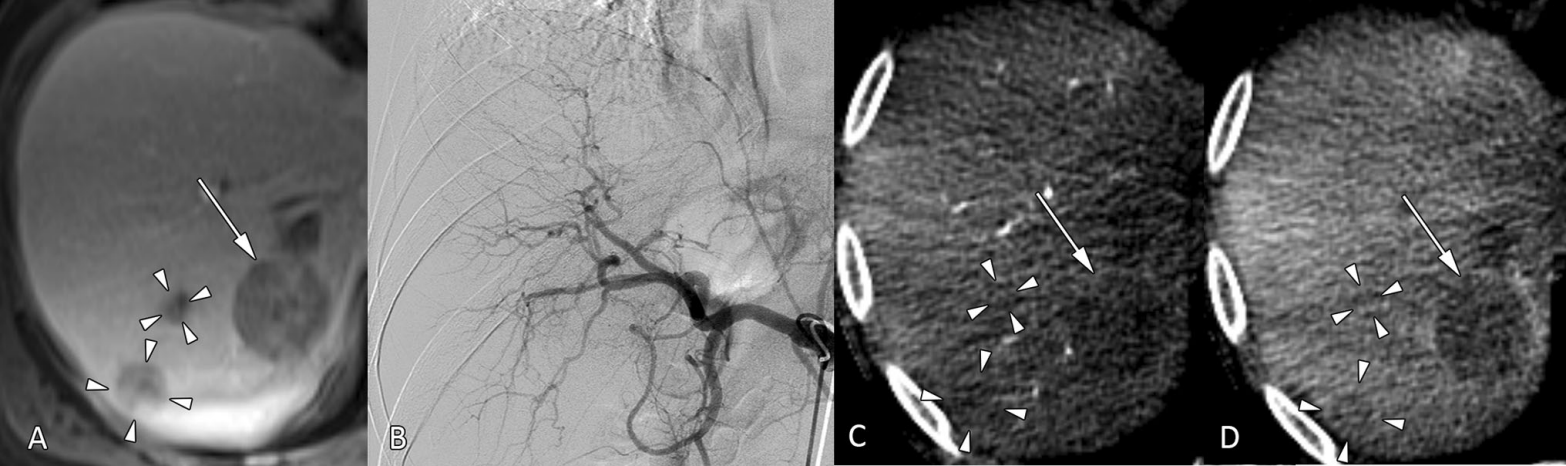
43-year-old man with a history of retroperitoneal sarcoma with liver metastases, treated using conventional TACE. A Contrast-enhanced T1-weighted gradient-echo sequence in the portal venous phase shows three lesions in segment 7, one larger (*arrow*) and two smaller tumors (*arrowheads*). B On the celiac arteriogram, none of the lesions is visible. C On early arterial phase CBCT, the large lesion is well depicted (*arrow*), but the two smaller lesions are difficult to distinguish (*arrowheads*). D On delayed arterial phase CBCT, all three lesions are well depicted (*arrow* and *arrowheads*)
